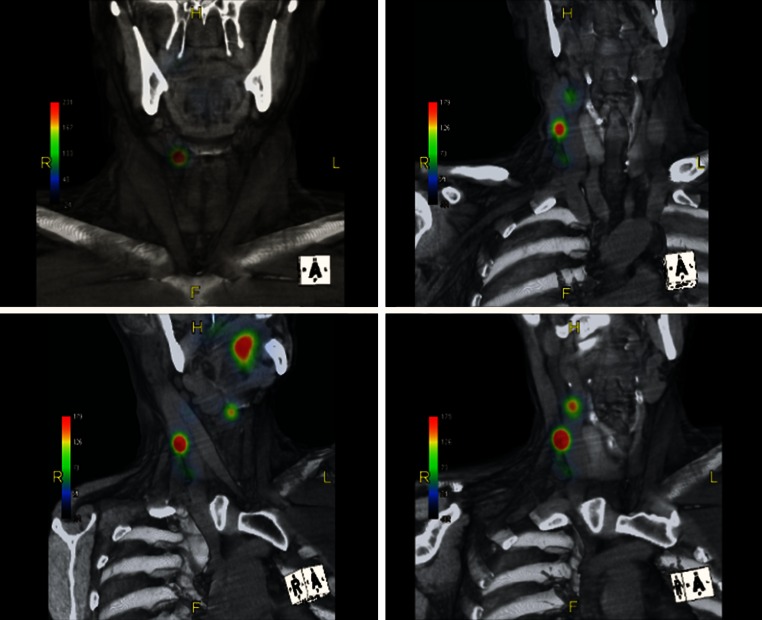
SPECT/CT three-dimensional fused reconstruction cross-sectional images of a typical patient with floor-of-mouth tumor (duration of SPECT/CT acquisition was 3–21 min post-injection of [^99m^Tc]tilmanocept. The *cube* in the lower right corner indicates the perspective of the image. *SPECT* single-photon emission computed tomography, *CT* computed tomography, *R* right, *L* left, *H* head, *F* feet, *A* anterior, *P* posterior
